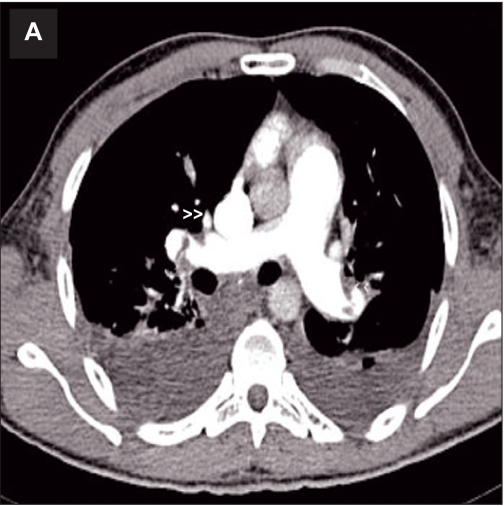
Computed tomography pulmonary angiogram showing right and left pulmonary artery emboli (A, arrow heads) and extensive thrombus in right pulmonary artery (B, arrow) in a patient with polycythemia rubra vera, splanchnic vein thrombosis and mobile right heart thrombus.
